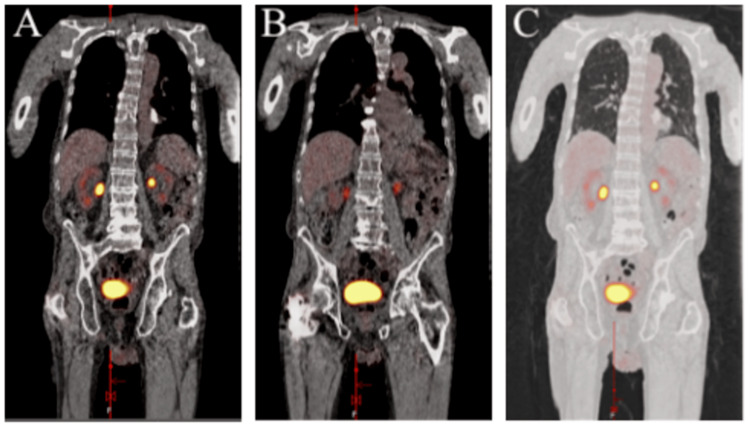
Positron emission tomography CT skull base to mid-thigh showing the absence of abnormal FDG avidity in the chest, abdomen, or pelvis. There are no solid lung lesions. CT: computed tomography; FDG: F-fluorodeoxyglucose
